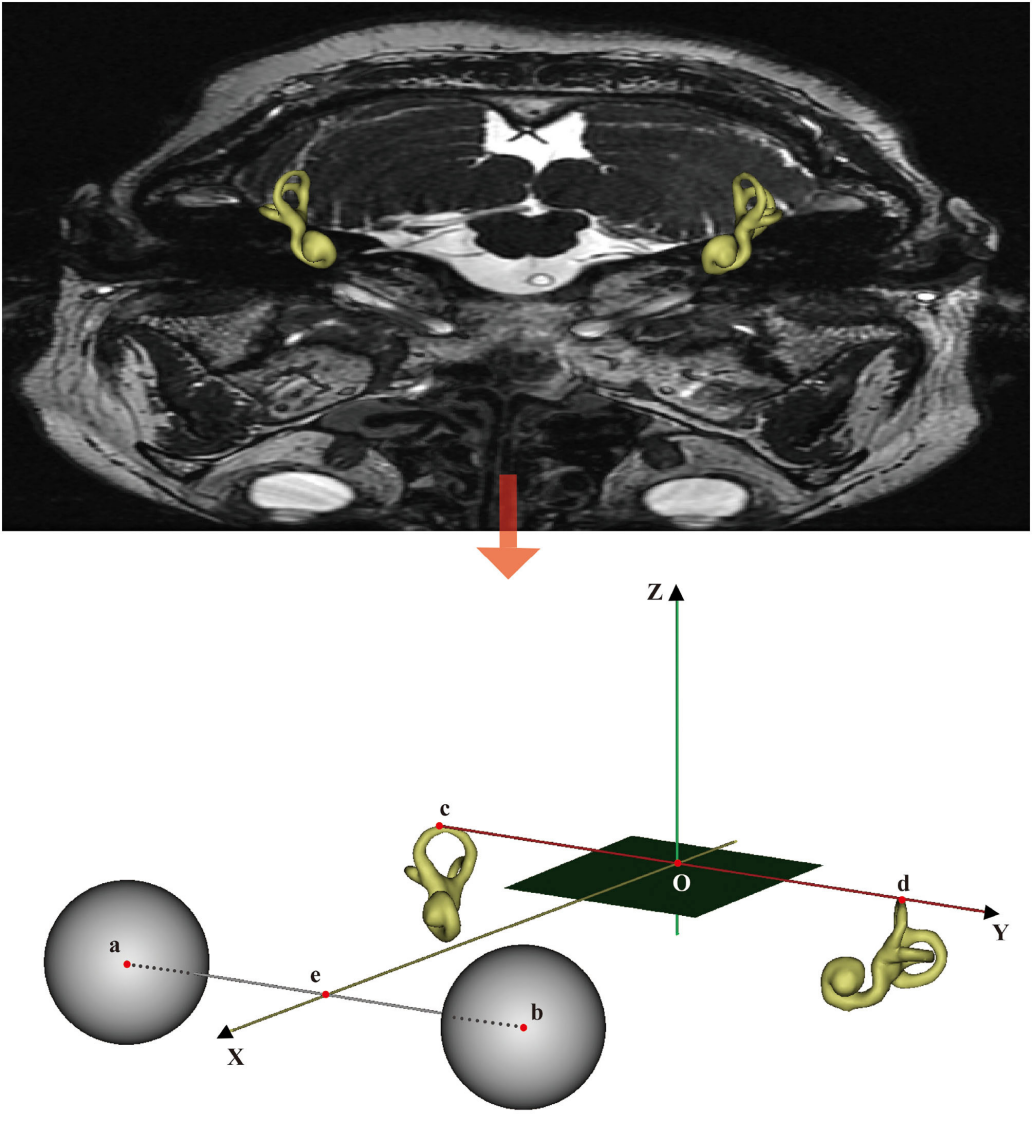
The W–X coordinate system overlaid on a CT image. Points a and b are the centers of the two eyeballs. Points c and d are the vertices of the bilateral superior semicircular canals. Point e is the midpoint of the line segment $\bar{\text{ab}}$. The origin of the coordinate system, point O, is the midpoint of line segment $\bar{\text{cd}}$. The *x-* and *y*-axes are defined by the rays $\vec{\text{Oe}}$ and $\vec{\text{Od}}$, respectively, and the *z*-axis is normal to the horizontal plane containing *x* and *y* (+superior). The SP is defined as the plane that contains point O and is perpendicular to the *y*-axis.
